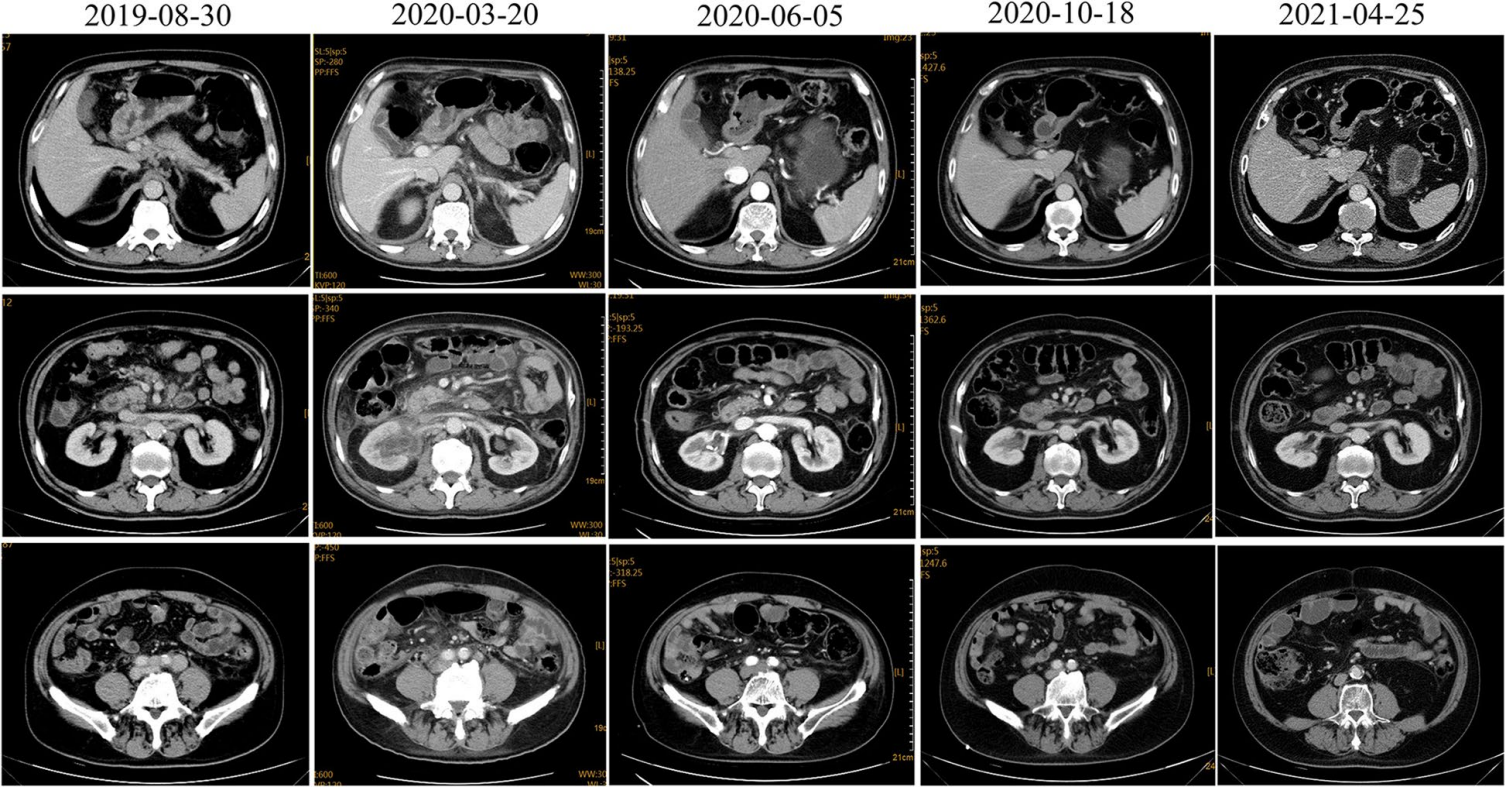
By regular CT scan, cancer in gastric antrum and metastases in other parts became progressively. Eventually, the tumor disappeared completely
